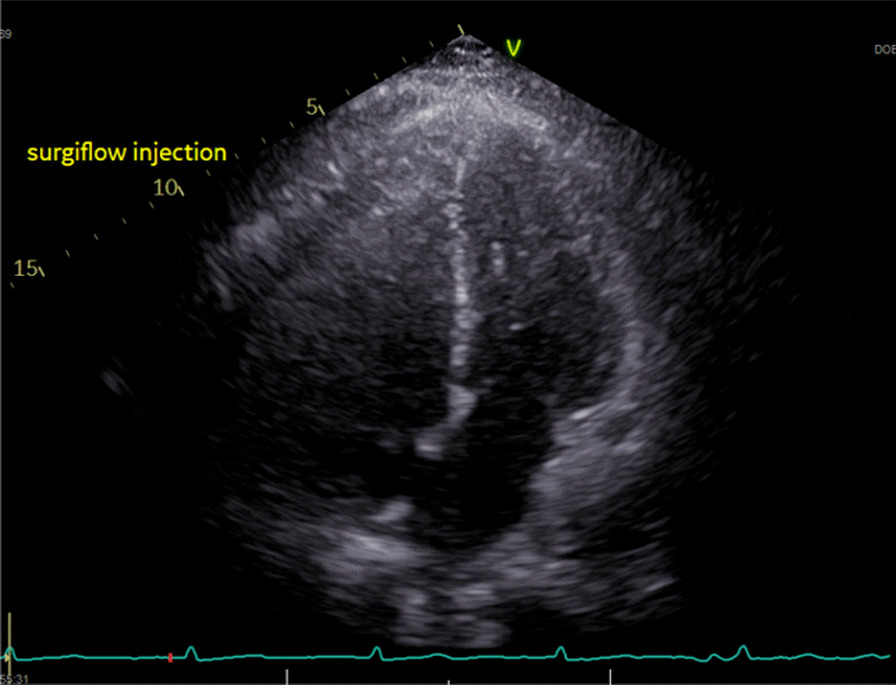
Patient 2 echocardiogram showing SURGIFLO Injection Through the Pericardial Drain. Note the Small but Present Effusion
